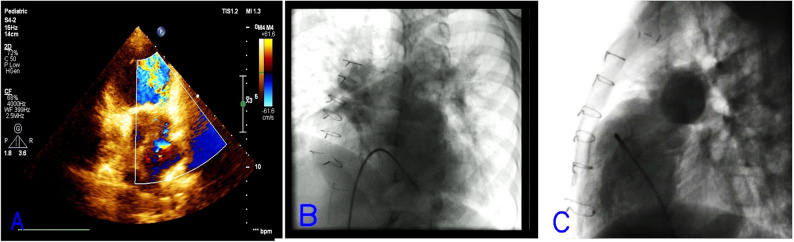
Preoperative diagnostic imaging. (A) Echocardiography showing a severe regurgitant jet indicating free pulmonary insufficiency. (B, C) Anteroposterior and lateral cardiac catheterization views demonstrating marked right atrial and ventricular dilatation
